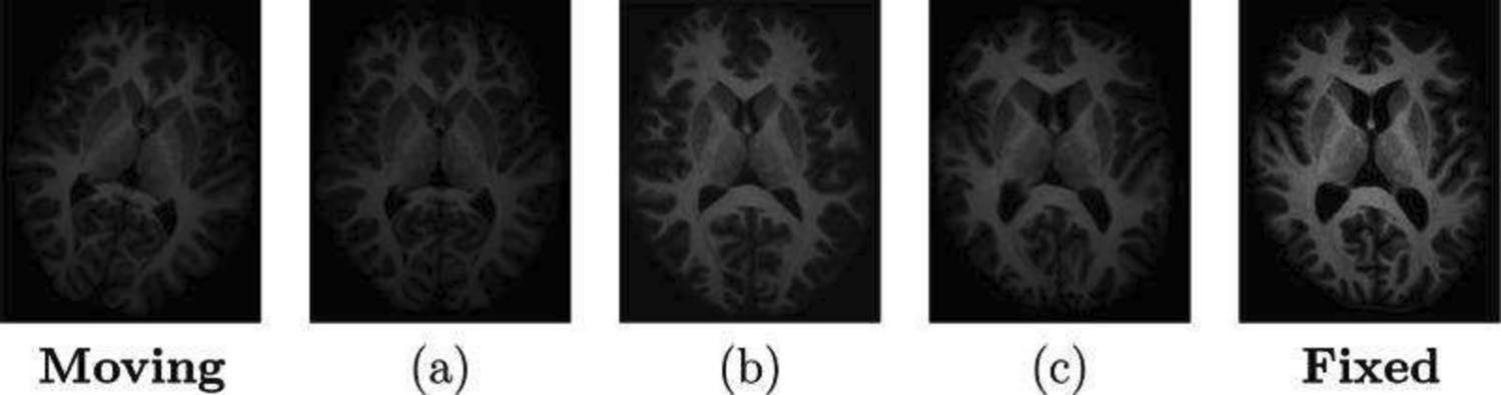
MRI images of the brain demonstrating the results of using three registration types between a moving and fixed image. Rigid registration was used in a, affine in b, and nonrigid/deformable registration in c. Image retrieved and modified from Chen et al. [4], 2024 Springer Nature
